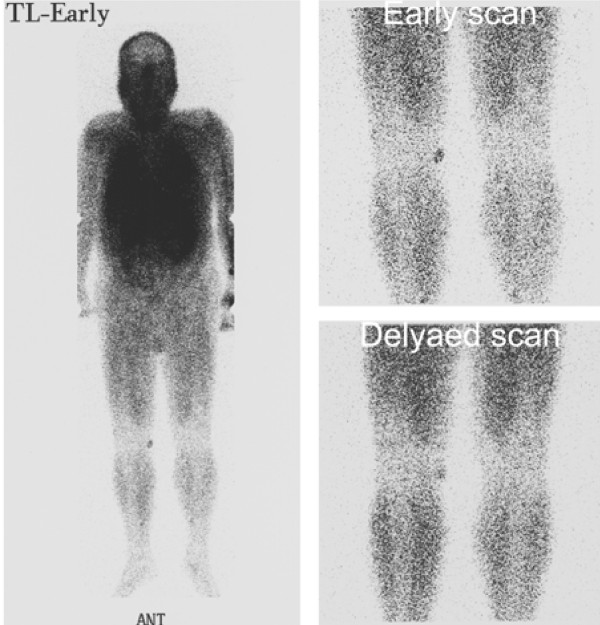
Tl-201 scintigraphy of the whole body at the early phase (left). Tl-201 was uptaken into the tumor in the right knee at the early scan (right upper) and continuously accumulated at the delayed scan (right lower).
